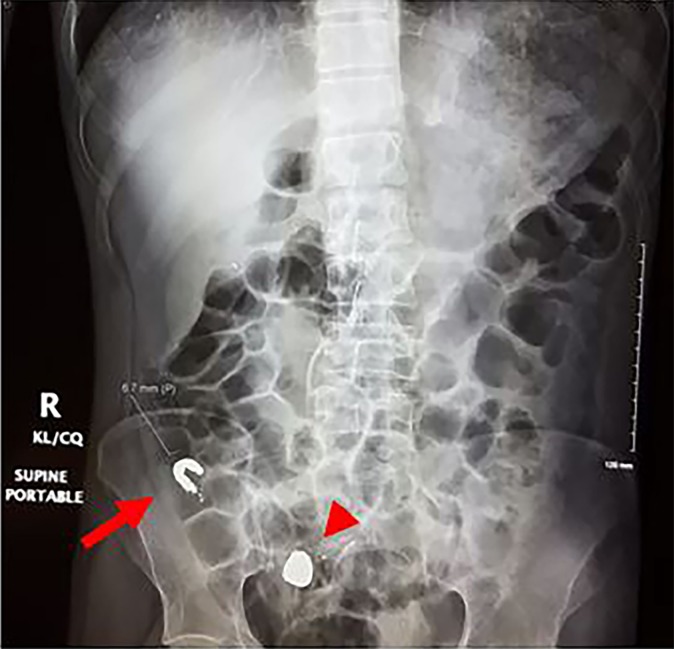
Abdominal radiograph demonstrating an interval new collection of the mercury in the right hemi-pelvis (red arrowhead) and interval decrease in the collection of mercury in the appendiceal lumen (red arrow).
